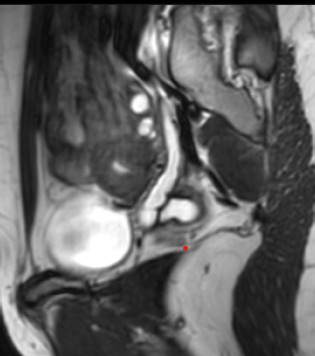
MRI showing a cystic tubular structure which is blind ending superiorly and coursing posterior to cervix, separate from the ovary.
